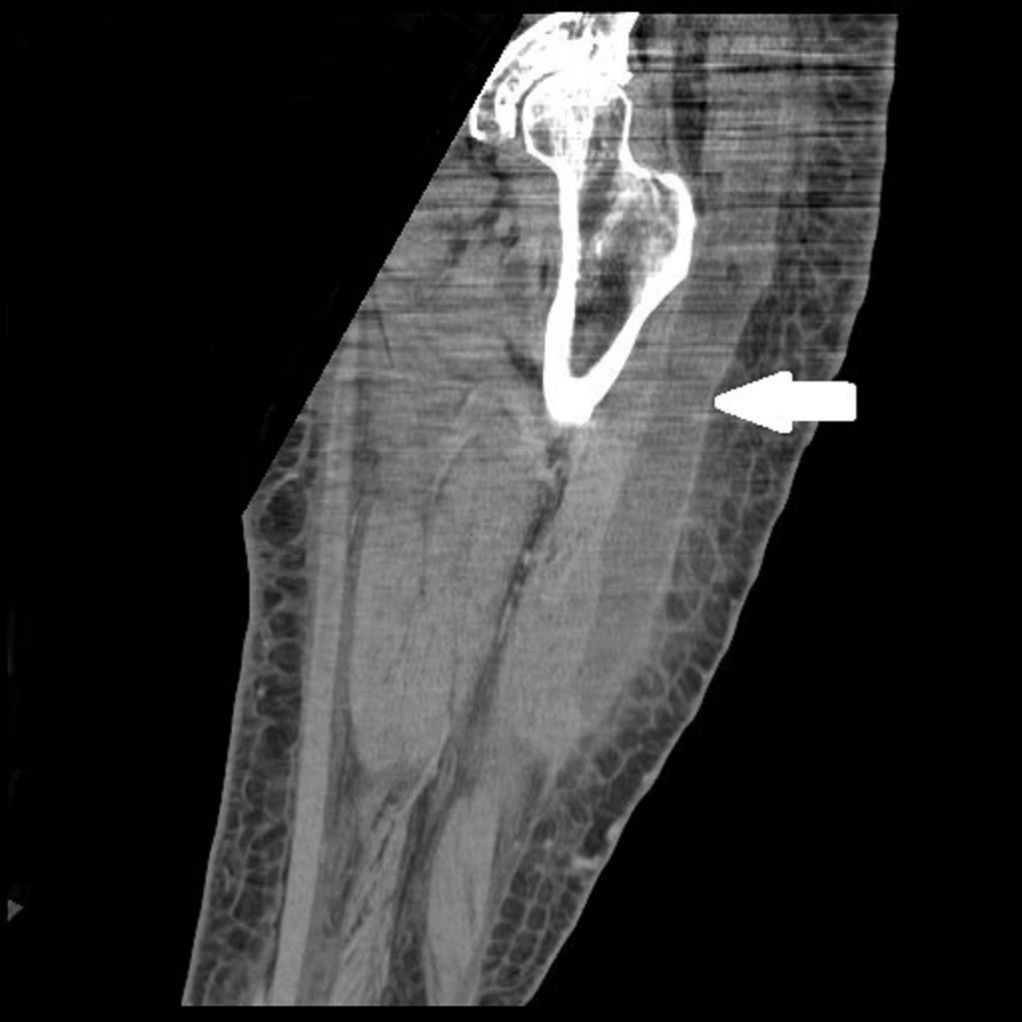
Coronal CT Scan of Left Lower Extremity Arrowhead demonstrates discrete intramuscular fluid collection with the largest focus centered in the vastus lateralis.
